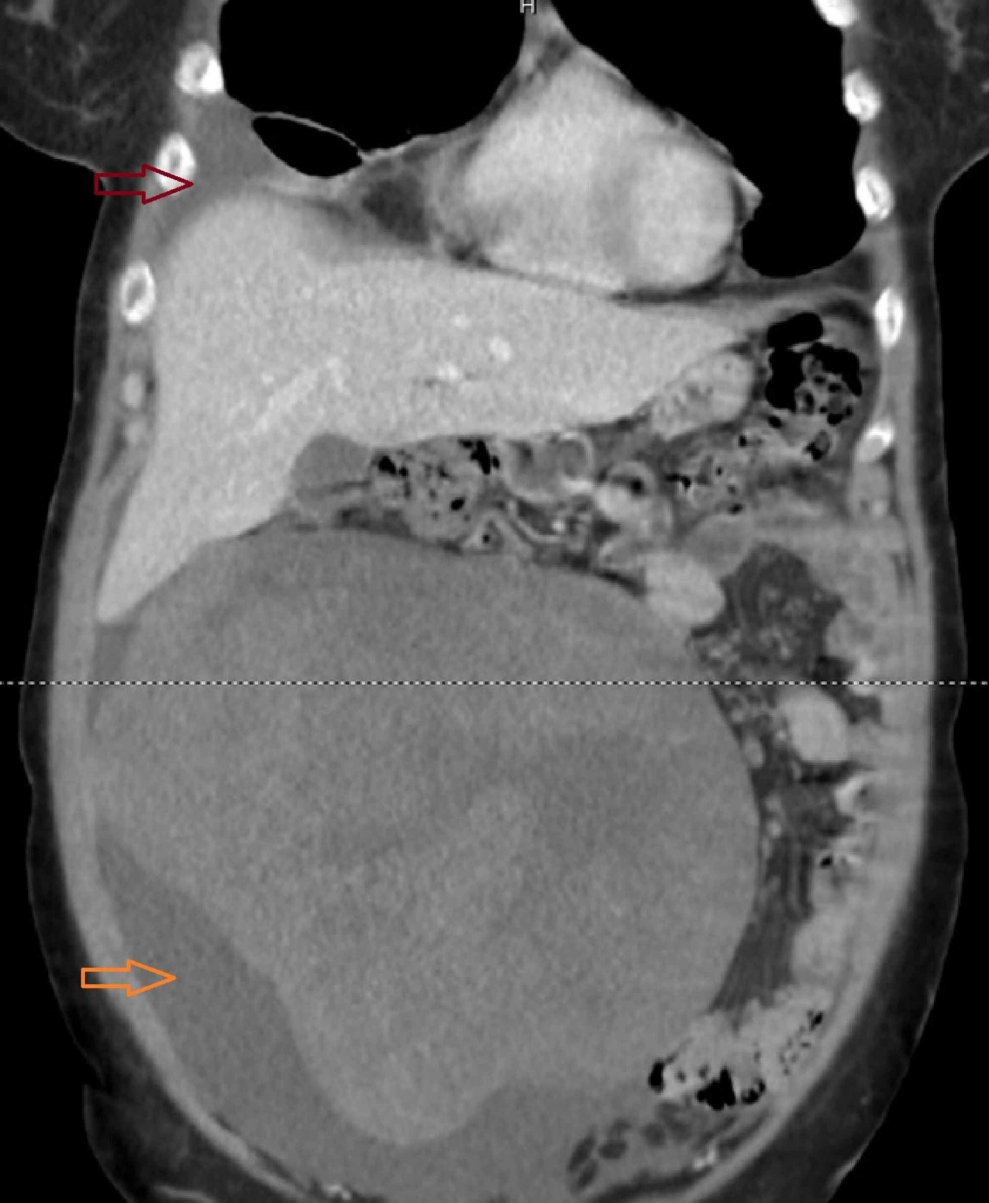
CT image, coronal view. A 21-cm abdominopelvic heterogeneous mass with clear borders and unclear origin – uterus versus ovary – is seen. Small amounts of peritoneal (orange arrow) and pleural fluid (red arrow) are observed. No lymphadenopathies were noted.
